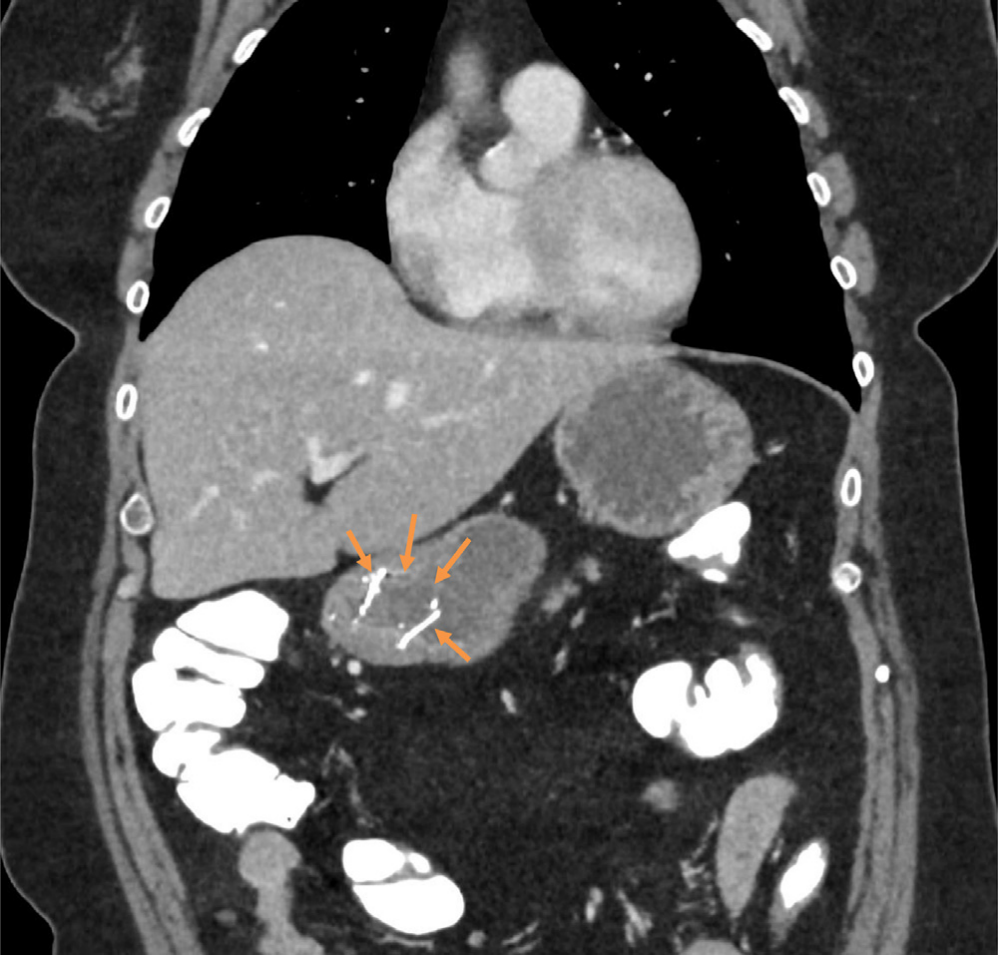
Stent fragment seen within stomach (arrows) on CT performed following contrast swallow.
